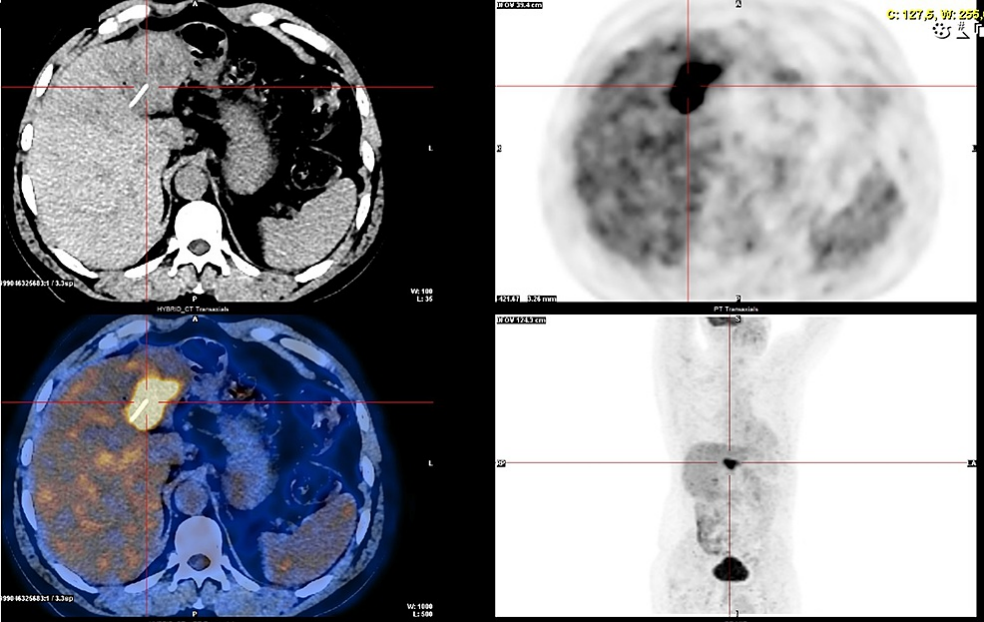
PET-CT consistent with secondary lesion. PET-CT: positron emission tomography-computed tomography
